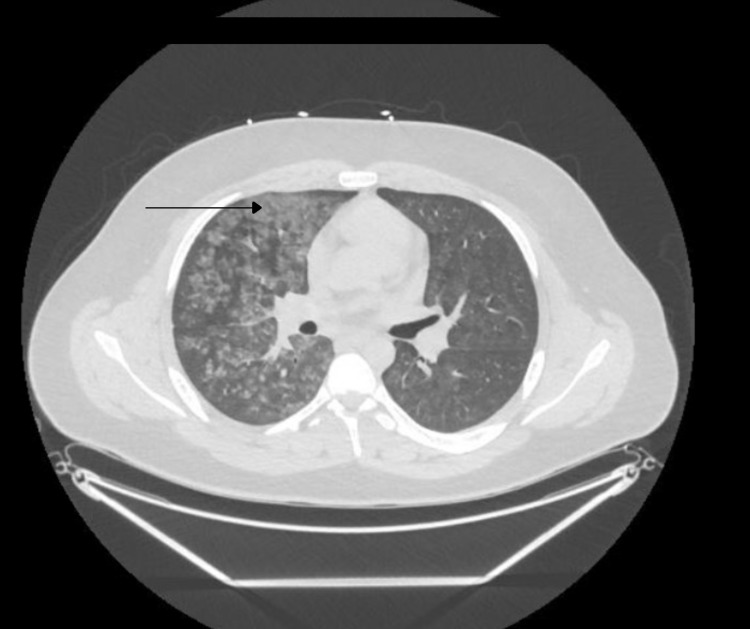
Chest CT scan with no contract showed diffuse ground-glass opacities (arrow) throughout the right lung and, to a lesser extent, in the left lung
